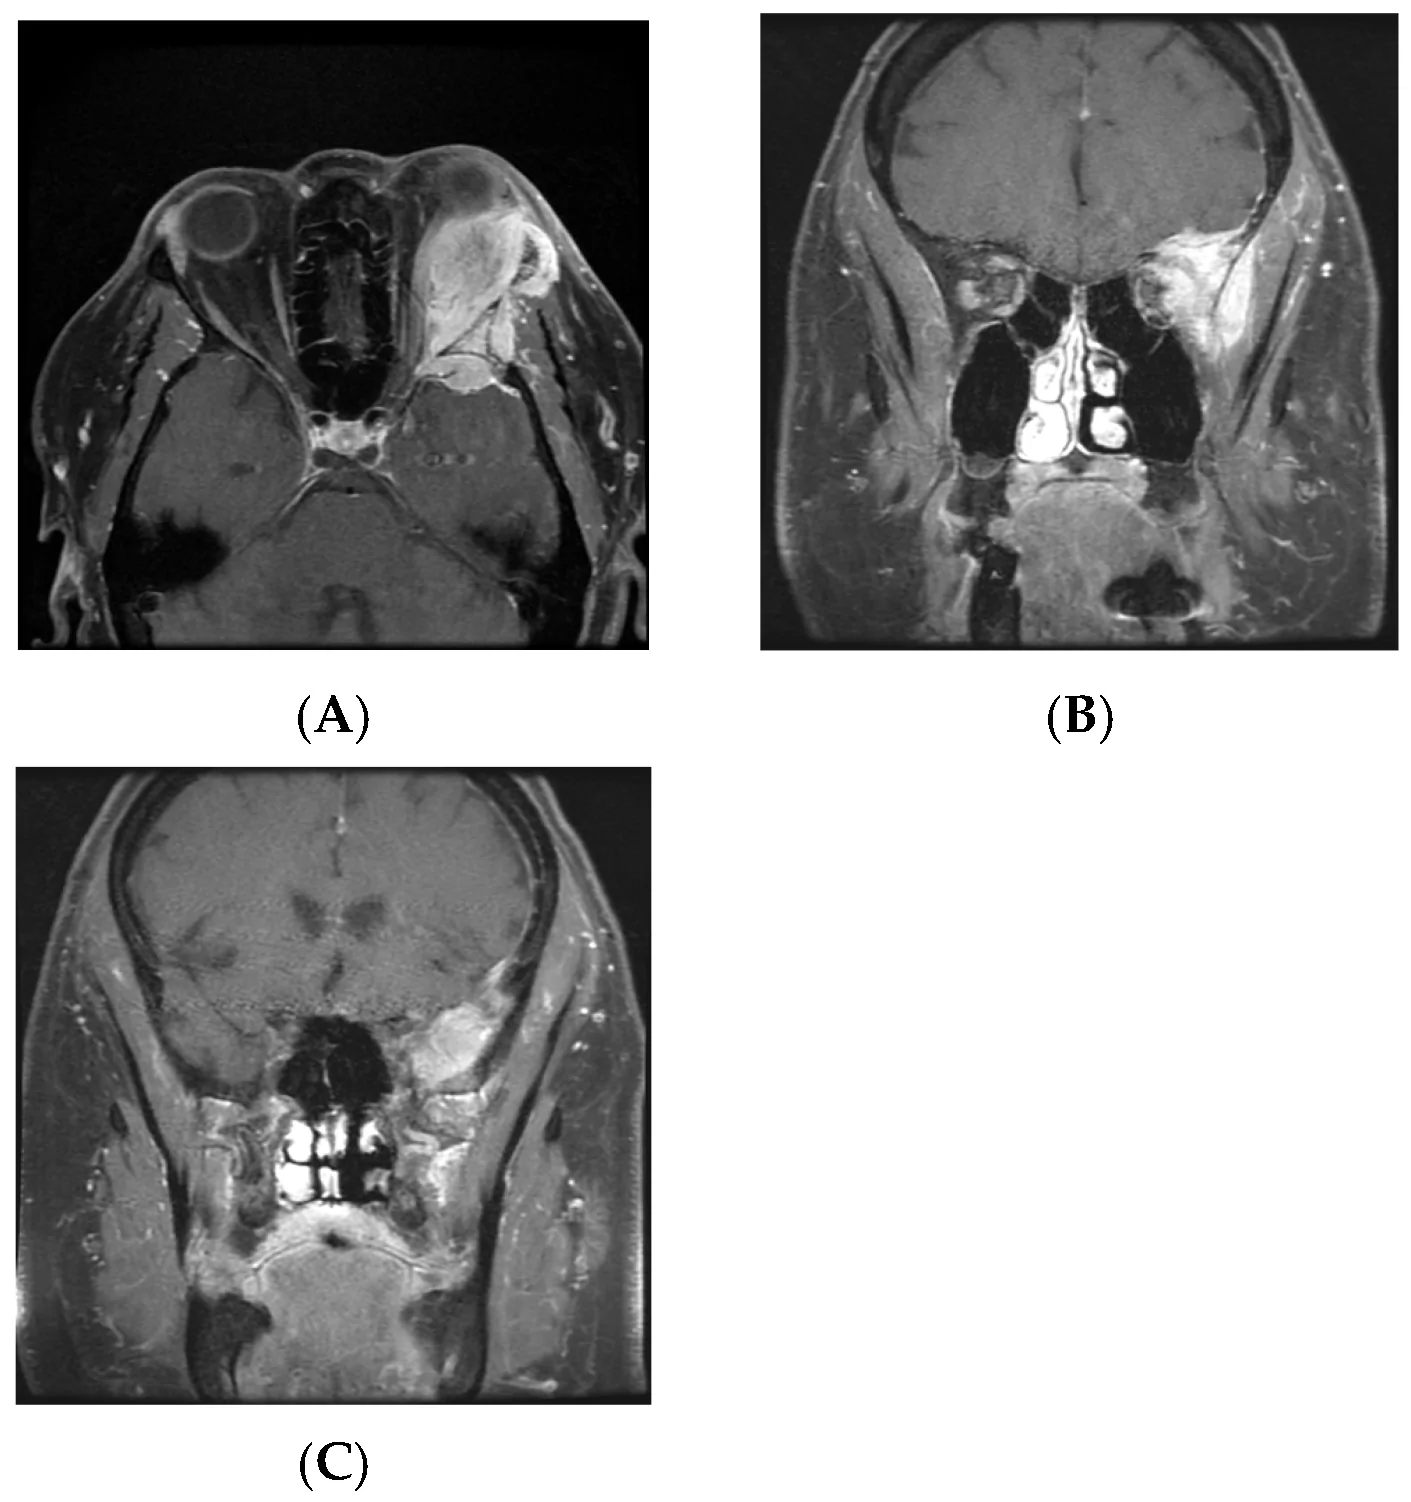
A 64-year-old man presented with progressive proptosis and complained of pain. An incisional biopsy prior to referral had confirmed the diagnosis of LGACC. (A–C) MRI findings on axial (A) and two coronal images (B,C) show a locally advanced adenoid cystic carcinoma of the left lacrimal gland with extension to the dura of the temporal lobe, sphenoid bone, and temporal fossa. Neoadjuvant intraarterial chemotherapy was given without any measurable response. The patient had an orbital exenteration through a combined frontal and cranial approach, with a modified orbital zygomatic craniotomy for approach to middle cranial fossa and infratemporal fossa with resection of middle cranial fossa tumor extension. He had a free flap reconstruction for the defect and adjuvant post-operative radiation therapy.
